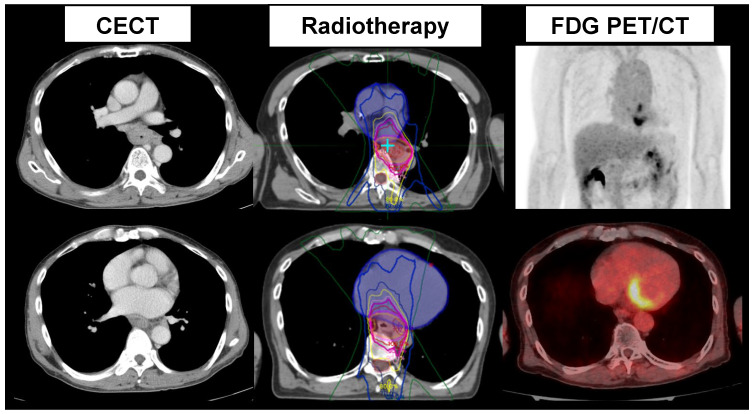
Contrast-enhanced CT (CECT) (left), radiation therapy planning (middle), and FDG PET/CT (right) after radiation therapy in a patient with esophageal cancer. High FDG uptake in the left atrium is noted, suggesting acute myocarditis after radiation therapy.
